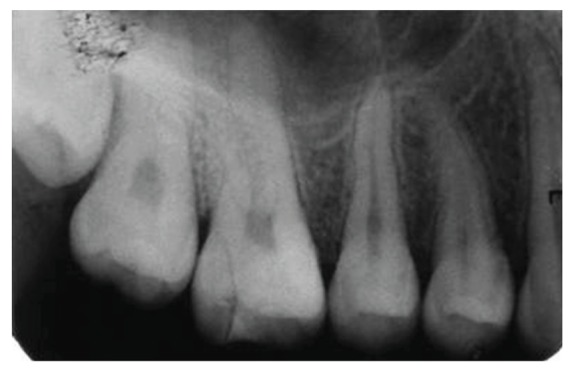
Preoperative radiograph revealing oblique fracture involving distal aspect of the tooth 16.
